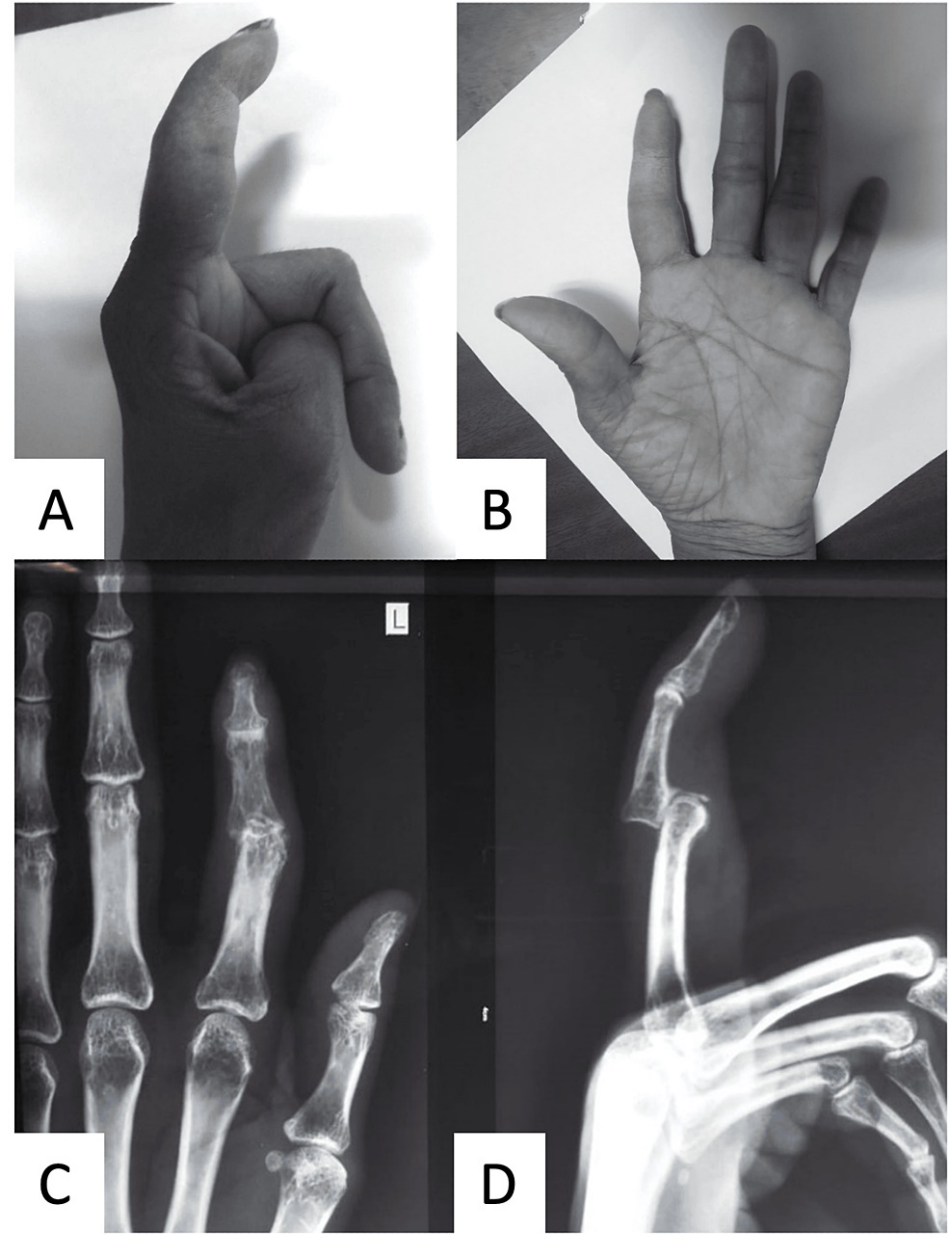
Figures A and B showing the pre-operative clinical image of the index finger with neglected PIP dislocation and Figures C and D showing the radiograph of antero-posterior and lateral projections of the index finger showing dislocation of the PIP joint. PIP: proximal interphalangeal joint
